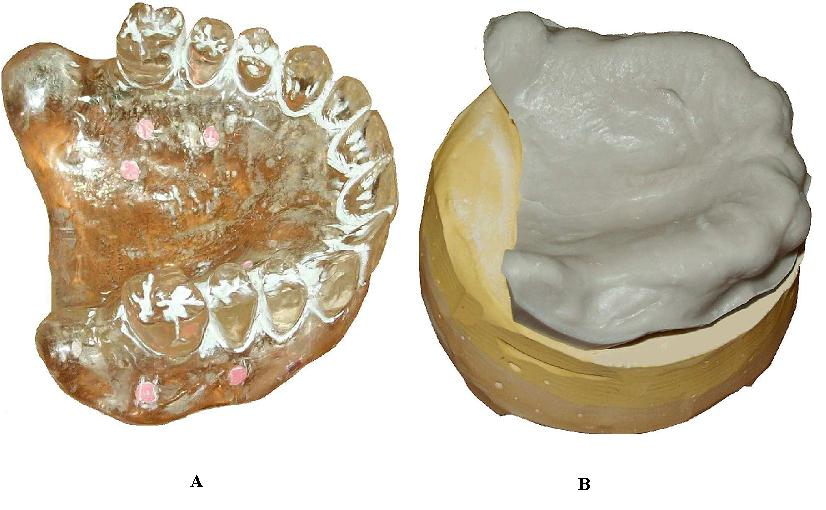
(A-B) Preoperative and anatomical dental models. (A) Radiographic template with gutta-percha markers, (B) gypsum dental cast.
